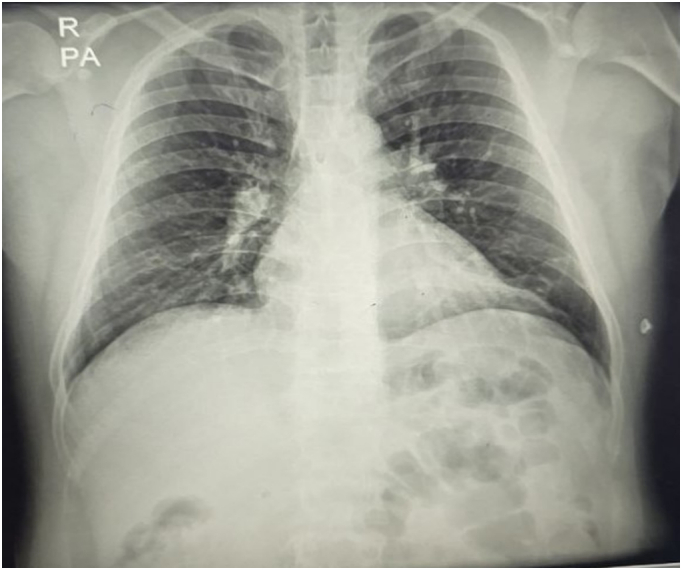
The chest X-ray image shows no abnormalities in the lungs or other organs.
